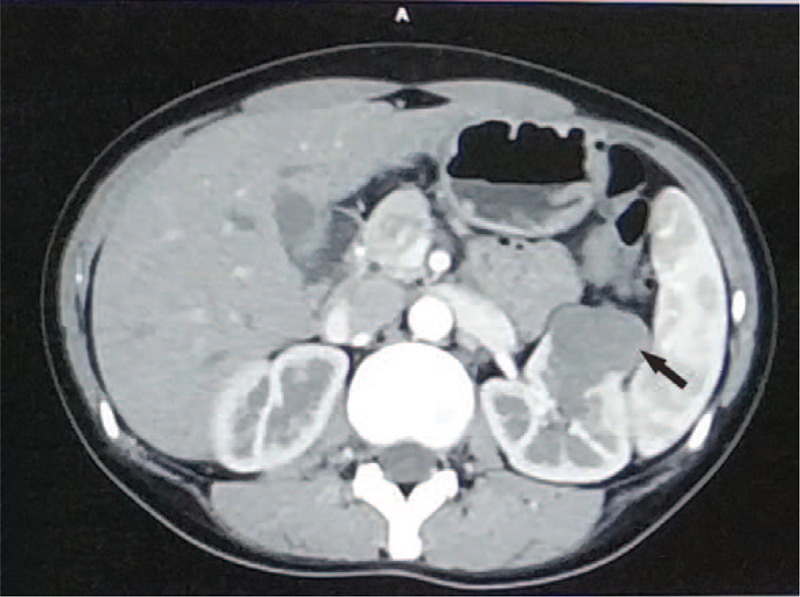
Abdominal pelvic enhanced CT. A round mass (black arrow) is showed in the middle part of the left kidney with a maximum diameter of 3.4 cm, slight enhancement in the early artery phase.
